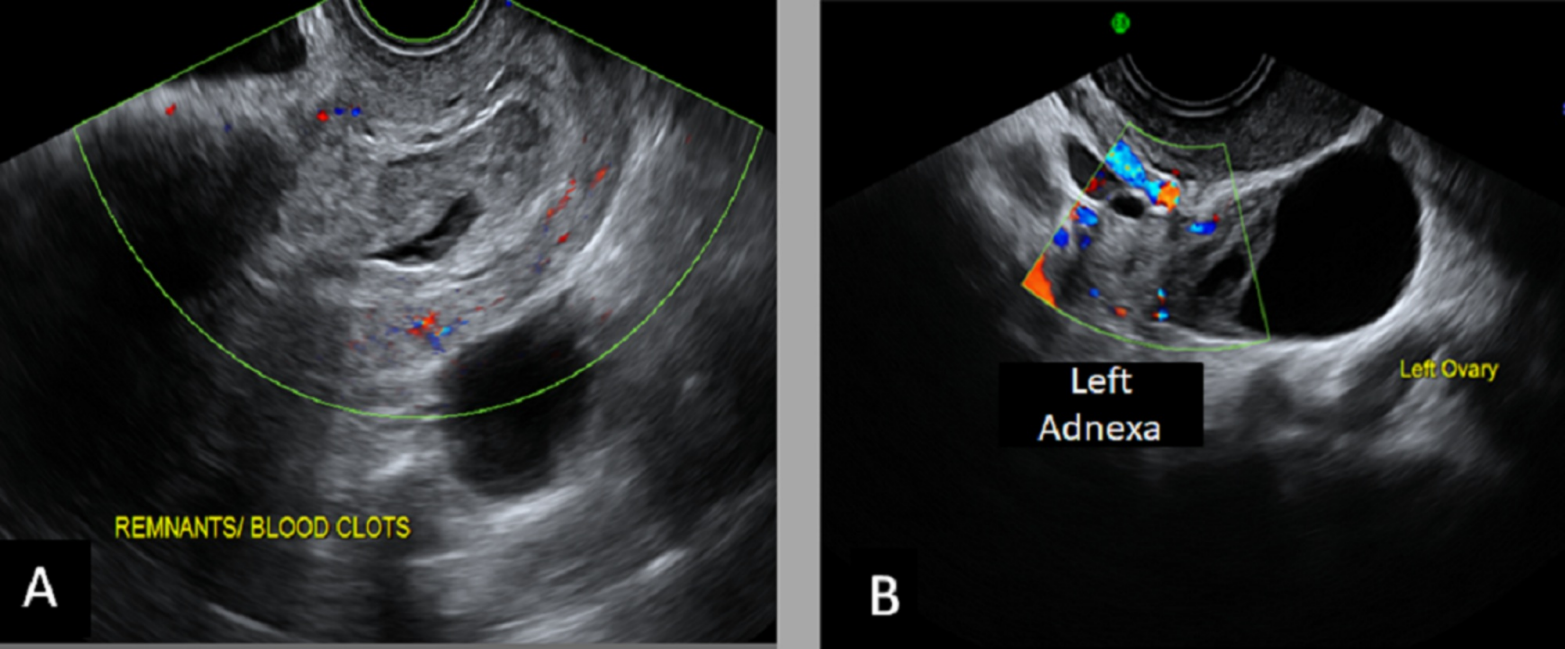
Transvaginal ultrasound A) There is an elongated sac-like structure seen in the lower uterine segment with a surrounding echogenic area (hematoma); B) A left adnexal echogenic mass is seen with peripheral vascularity noted by a color Doppler study. These findings are in favor of heterotopic pregnancy with the incomplete abortion of the intrauterine pregnancy.
